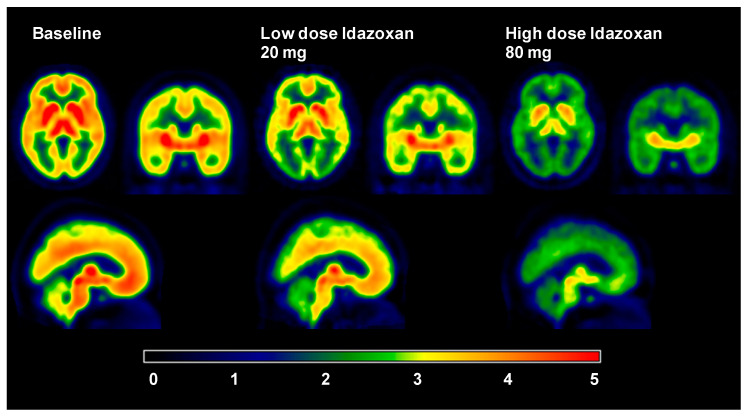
SUV images for healthy volunteers which demonstrates the heterogeneous brain uptake in all regions and dose-dependent blockade by idazoxan (p.o.) of ^11^C-BU99008 (Taken from [9]).
